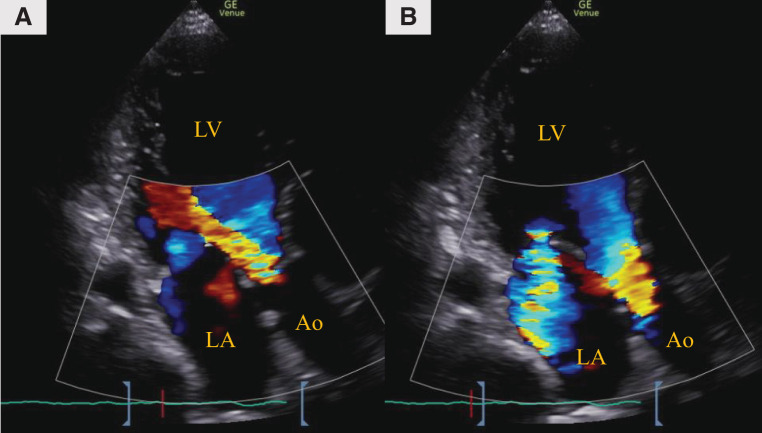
Preoperative transthoracic echocardiography. (A) Aortic valve regurgitation and (B) Mitral valve regurgitation. Ao, aorta; LA, left atrium; LV, left ventricle
